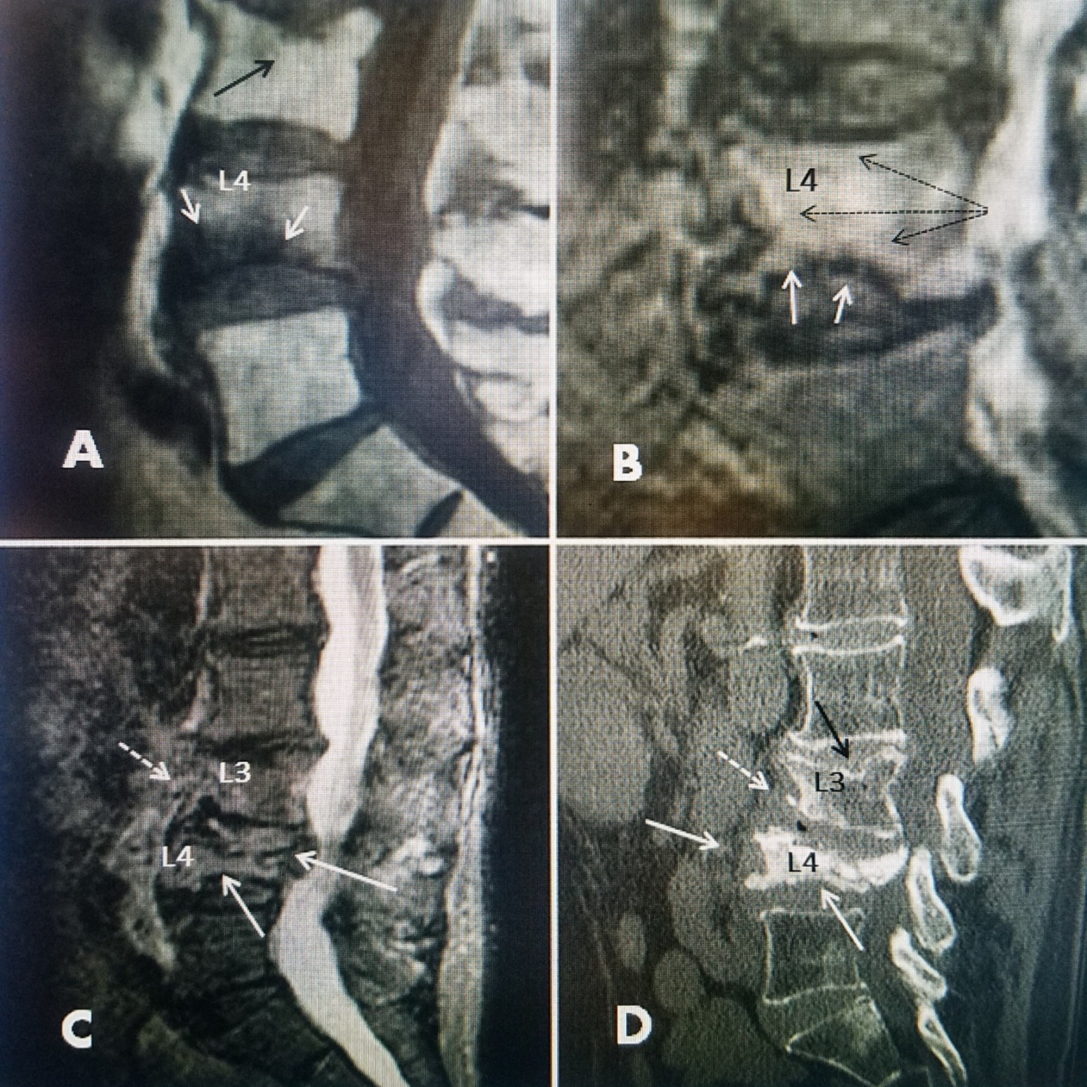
Progressive collapse of an inferior L4 endplate fracture over nine months A: Initial T1 magnetic resonance imaging (MRI) three weeks after a fall, showing a small, inferior endplate fracture at L4 with 50% vertebral body edema (solid white arrows). There is an old, small superior endplate fracture at L3 (solid black arrow). B: The patient used a lumbar support for three months but because of persistent pain, a repeat MRI scan was performed four months after injury and a short TI inversion recovery (STIR) MRI showing almost 100% edema of the L4 vertebral body indicated by a high-intensity uptake extending throughout L4 (dotted black arrows). The main area of the fracture is confined to the inferior endplate (solid white arrows) although the edema is spread in the entire L4 vertebra. Patient refused vertebral augmentation at that time. C: MRI scan seven months after injury now showing the progressive collapse of L4 with the posterior protrusion of the vertebra into the lumbar canal. There is also a "mottled" marrow single in L3 (dashed white arrow), suggesting a new adjacent-level fracture. D: Patient progressed to a "pancake" like collapse. Bilateral vertebral augmentation (VA) was performed to stabilize the fracture and the patient had an 80% reduction in pain after two weeks. However, the patient is developing a new endplate fracture inferiorly at L3 (dashed white arrow). The old superior endplate fracture initially seen when first diagnosed is still apparent (solid black arrow). Biopsy performed at the time of vertebral augmentation (VA) was negative for cancer and only showed severe osteoporotic bone.
